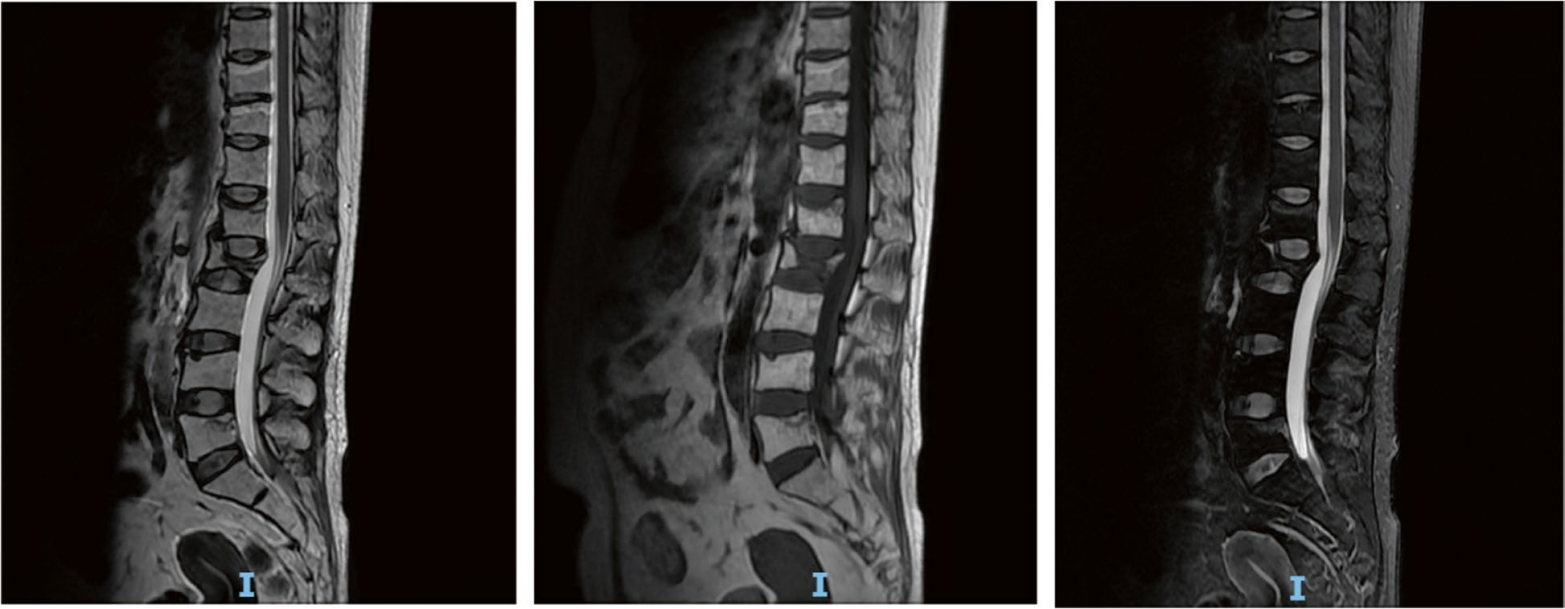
The MRI of lumbar spine showed the occurrence of new vertebral compression fracture in first lumbar vertebra duding hospitalization in the department of urology
